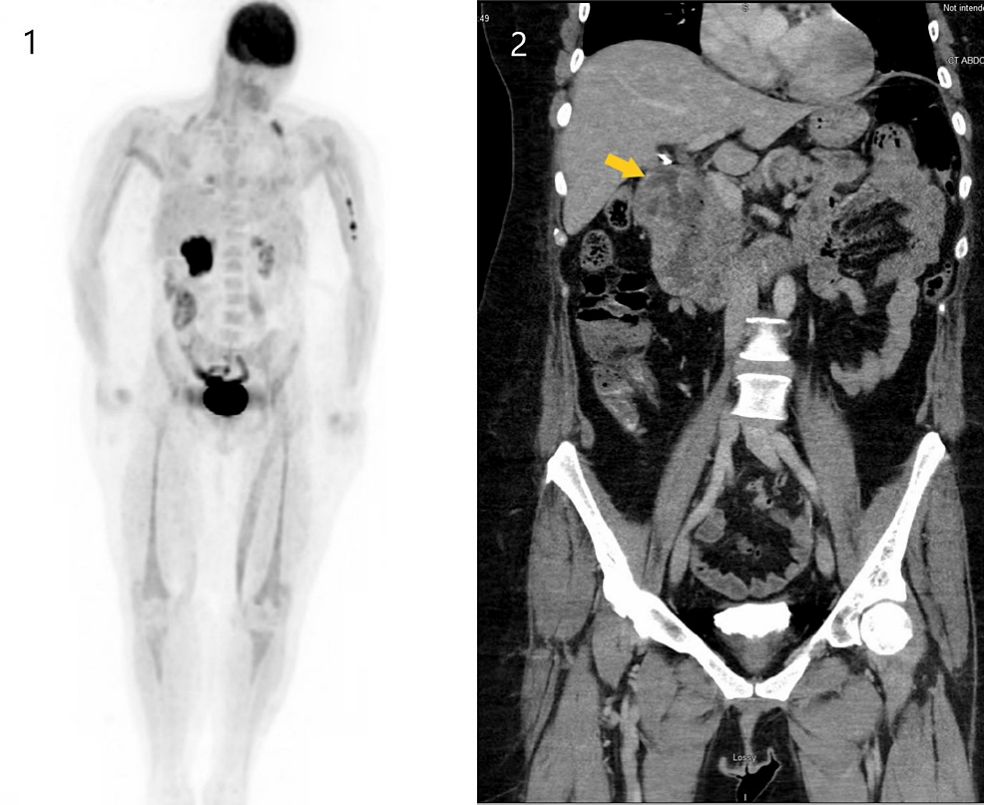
PET scan (1) and anteroposterior CT of the abdomen and pelvis (2) showing inflammation and increased uptake in the duodenum
